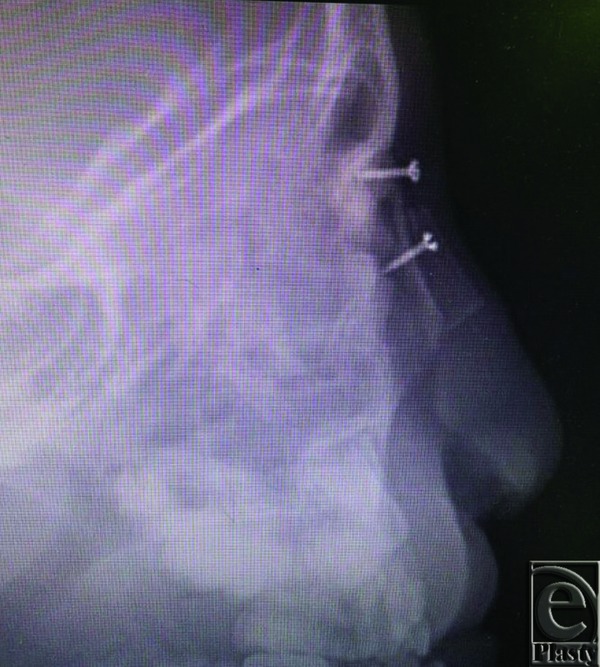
X-ray film showing the position of the screws used for fixation of the graft.
